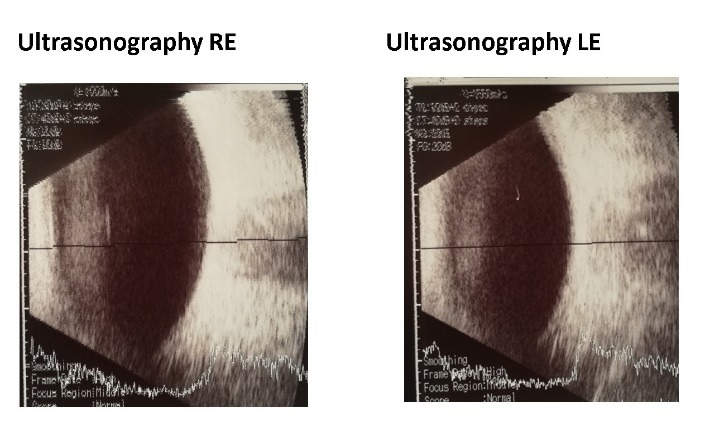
Ultrasonography of the right and left eye
